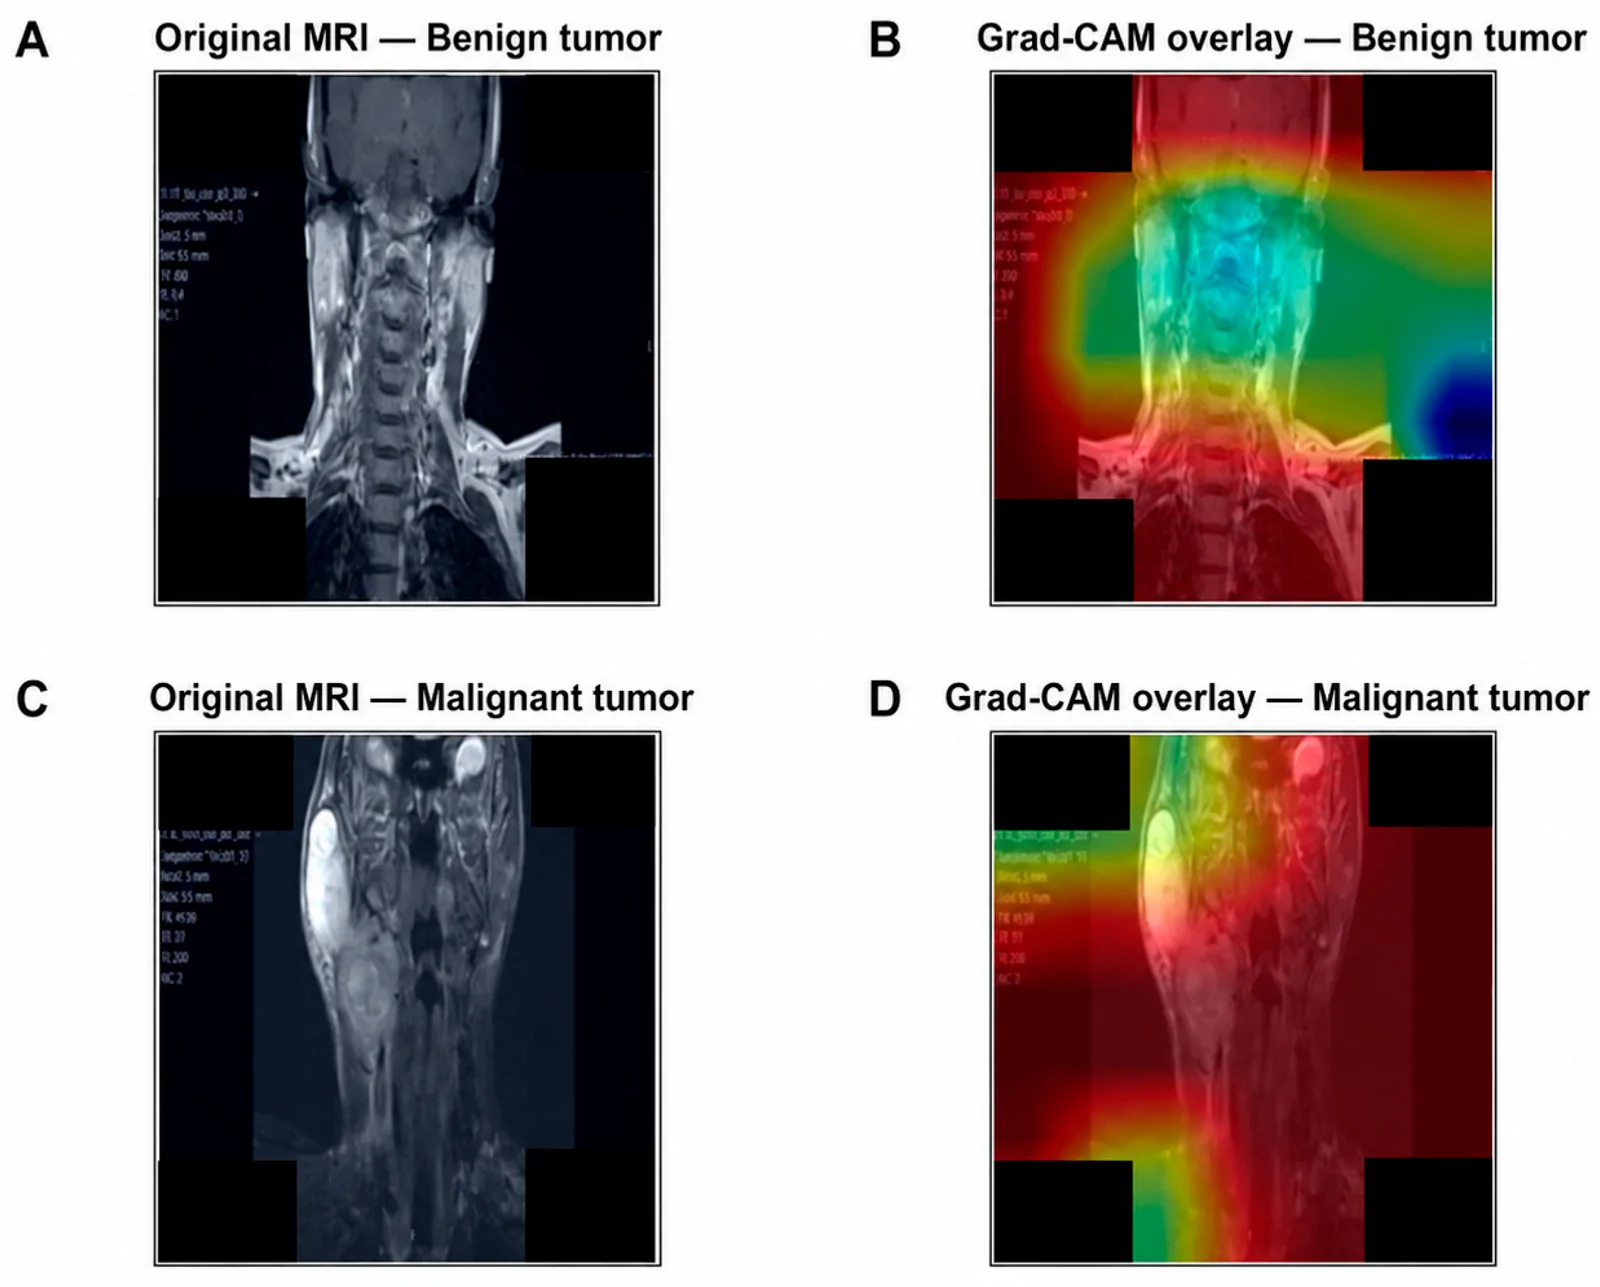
Representative Grad-CAM visualizations of benign and malignant parotid tumors. Panels (A,C) show the original MRI images of representative benign and malignant tumors, respectively, with the lesions indicated by arrows. Panels (B,D) show the corresponding Grad-CAM overlays. Warmer colors indicate image regions with greater contribution to the model prediction. Grad-CAM was used for qualitative interpretability and should not be considered a tumor segmentation or localization method.
